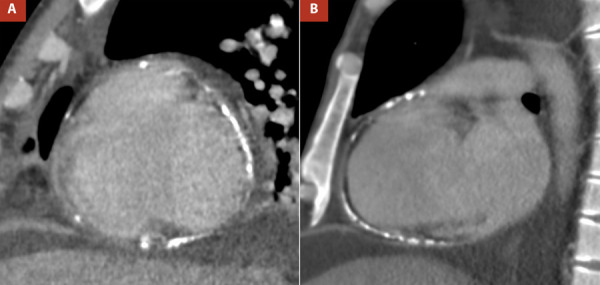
A,B Thoracic radiography, anteroposterior view, showed pulmonary congestion and right pleural effusion, C. Non-contrast non-gated computed tomography reformat revealed thickened pericardium (3.7 mm) with diffuse calcification, D. Computed tomography sagittal reformat demonstrated calcified pericardium involving right chambers.
